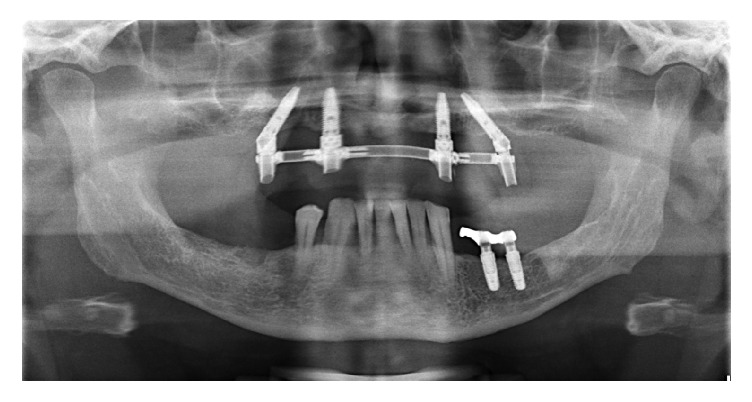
Panoramic radiograph at 60-month follow-up.
